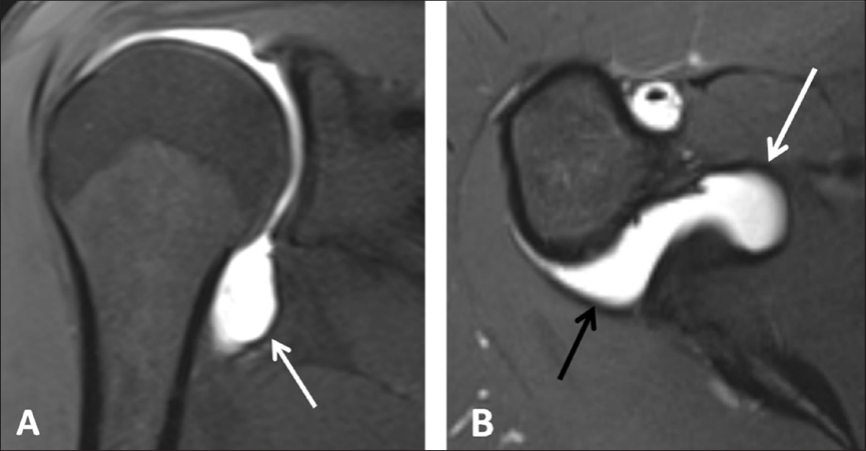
Inferior glenohumeral ligament. On fat-saturated T1-weighted MRA images obtained in (A) Coronal oblique and (B) Axial planes, the ligament appears as a thin hypointense band delimited by the distended axillary pouch or recess with a U-shaped appearance (arrow, A). The anterior (white arrow, B) and posterior (black arrow, B) bands are demonstrated on the axial section.
